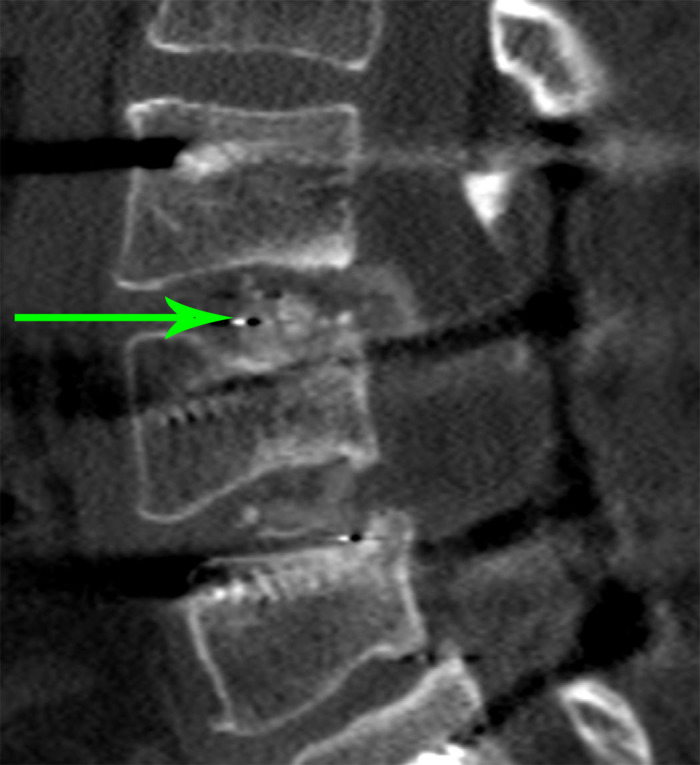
Cage Fracture and Migration CT imaging reveals a fracture of the interbody cage and its subsequent migration into the spinal cord.
